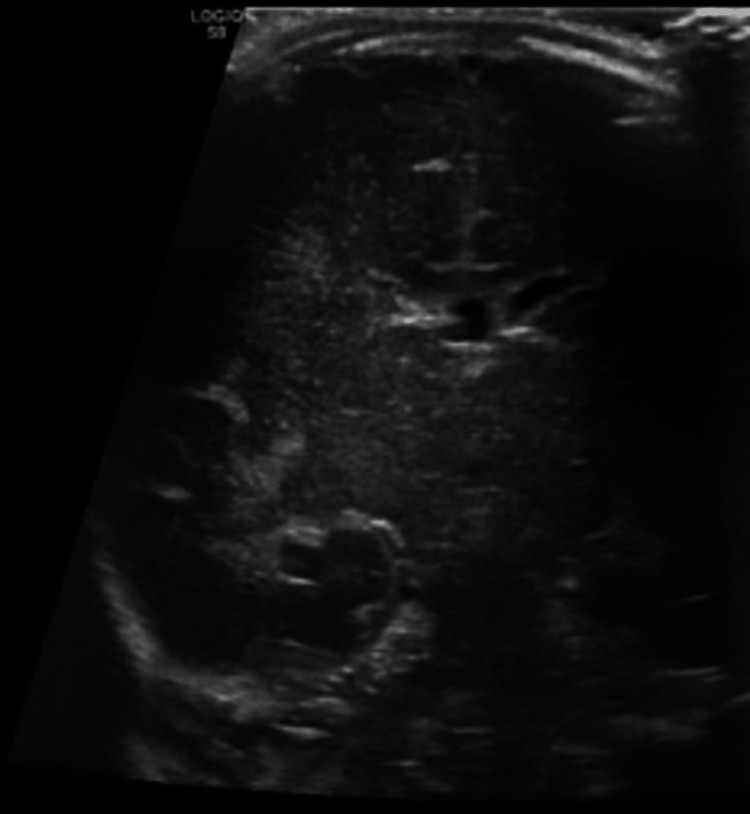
Transcranial ultrasound (20 minutes later) showing improvement in gray-white matter interface and the size of ventricles, decreased hypoechoic foci.
